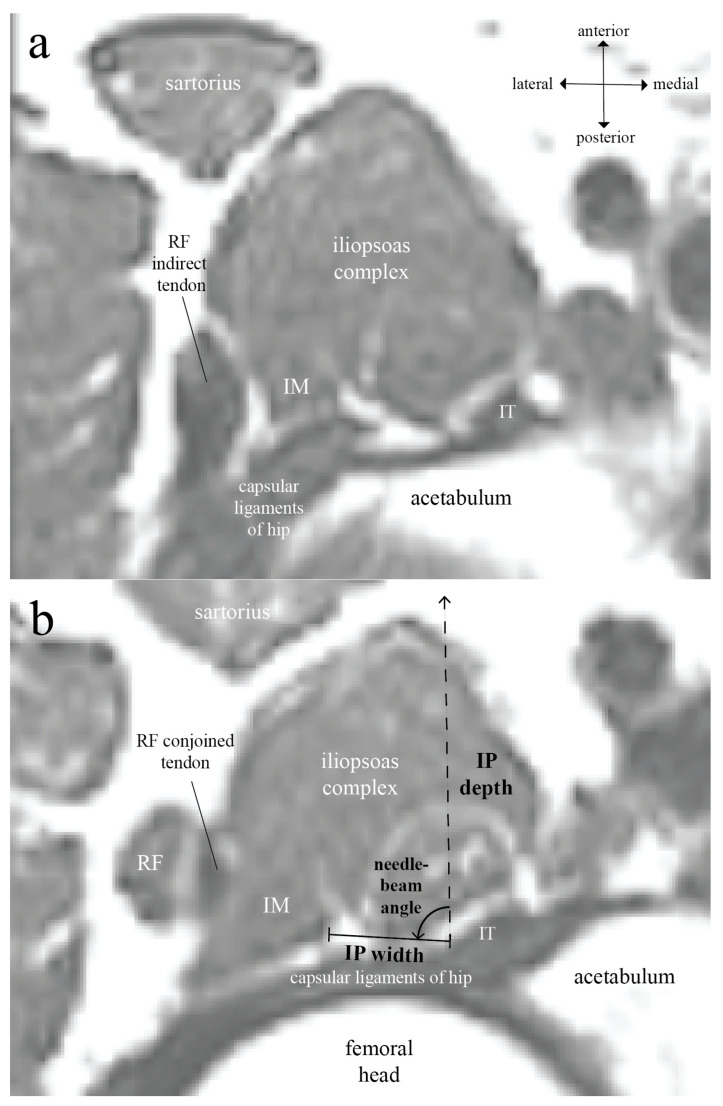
Axial plane of T2-weighted magnetic resonance (MR) images of the human right hip: (a) At the level where rectus femoris (RF) indirect tendon appears and extends anteroposteriorly towards acetabular rim; (b) At the level 10–15 mm caudal to Figure 1a, where the RF indirect and direct tendon merge into its conjoined tendon, the iliopsoas plane (IP) width, depth, and needle-beam angle were measured. The anterior skin surface used for IP depth measurement is not shown.
